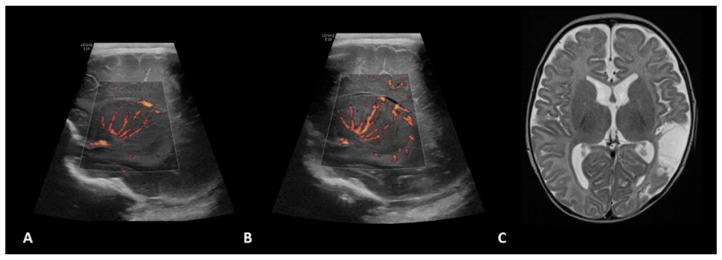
A 1.5-month-old girl with a history of transposition of the great arteries (TGA) underwent ECMO due to failure to wean from cardiovascular bypass after major cardiac surgery. Brain MVI was performed after three days from cannulation; grayscale US (not shown) demonstrated a small focus of increased echogenicity within the left caudate. Right (A) and left (B) parasagittal brain MVI showed mild bilateral tortuosity of the lenticulostriate vessels. The patient remained on ECMO for a total of four days and presented with seizures two days after decannulation, secondary to a cardioembolic stroke in the territory of the posterior left middle cerebral artery, as shown in axial T2-weighted MRI (C) obtained two months later.
